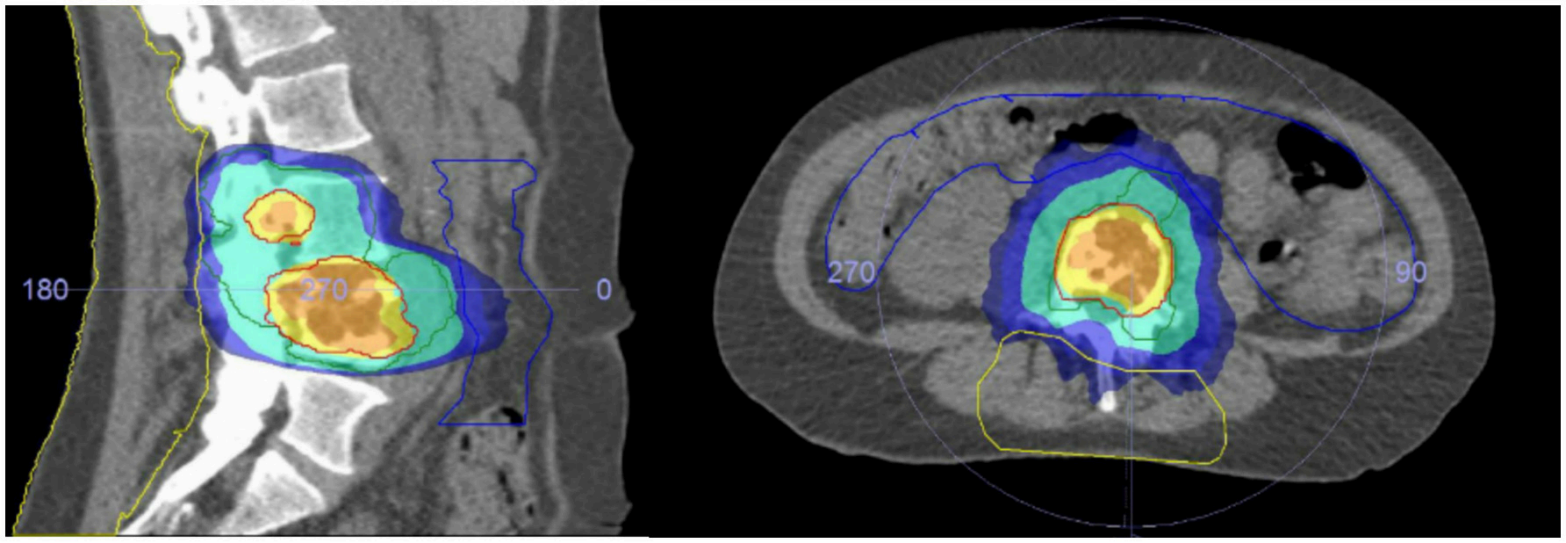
Planning CT showing a L4 metastases. The surgical area is depicted with the yellow lines. The dark blue area receives 8Gy, the turquoise area 9Gy, the yellow area 16.2Gy, and the orange area 18Gy.
